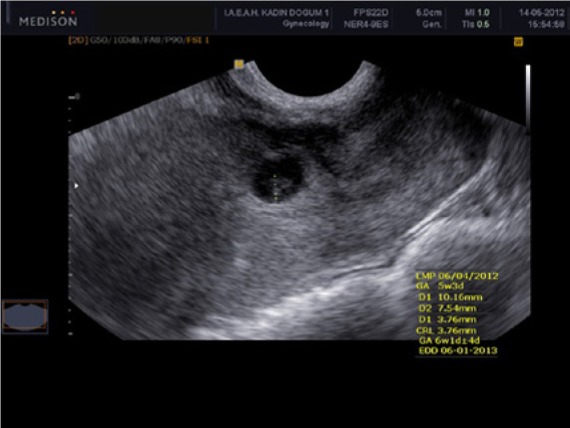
Ultrasound examination of case 2.
